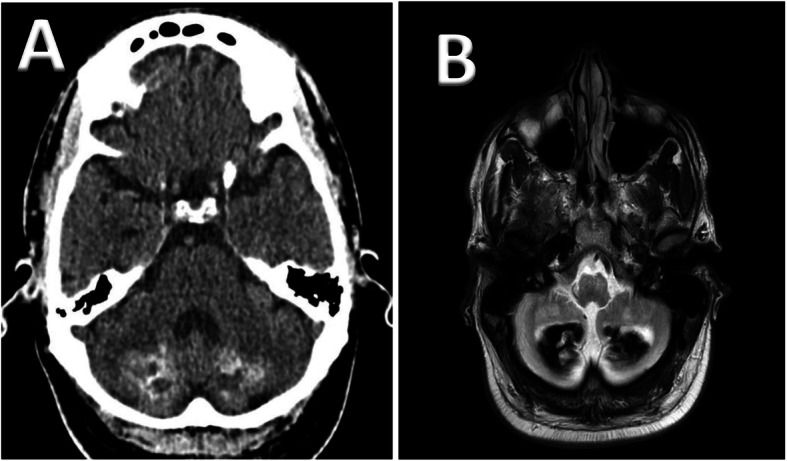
CT (a) and MRI (b) images from Patient 3 showing evidence of cerebellar calcification which although rare can be a feature of CTX
